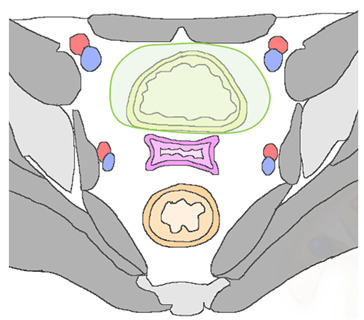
• On T2WI: o In the bladder: intermediate-to-high SI soft-tissue mass interrupting the normal low-signal-intensity of the bladder wall (probable muscle invasion), or the normal high SI of the mucosal layer (mucosal involvement). Vesicovaginal fistula appears as a linear fluid-filled tract, with disruption of the posterior bladder wall and infiltrative soft tissue into the bladder lumen or in peri-vesical space. o In the urethra: disruption of this bullseye aspect. • The use of post-contrast images and DWI may help differentiate bladder edema from true tumor infiltration. On post-contrast images fistulous track may be seen as linear peripheral enhancement connecting adjacent structures. • On excretory-phase CT scans contrast material may spread from the filling urinary bladder into the vaginal cuff or vagina. Central involvement of the bladder trigone at the ureteral orifice can cause ureteral obstruction, resulting in hydronephrosis.
